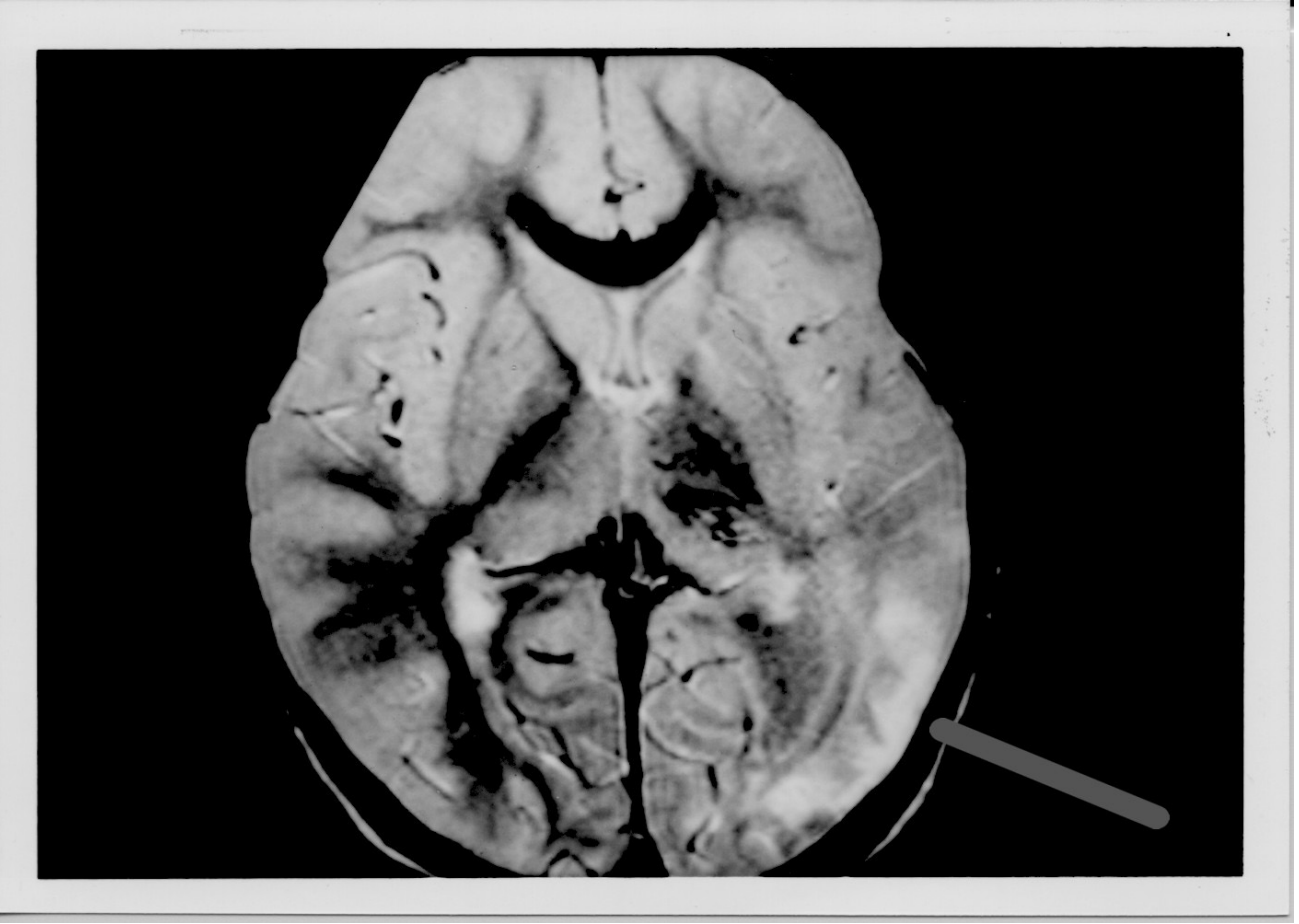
Magnetic resonance imaging obtained the day after admission demonstrating acute ischemia in the left temporal and parietal area (horizontal gray bar).
